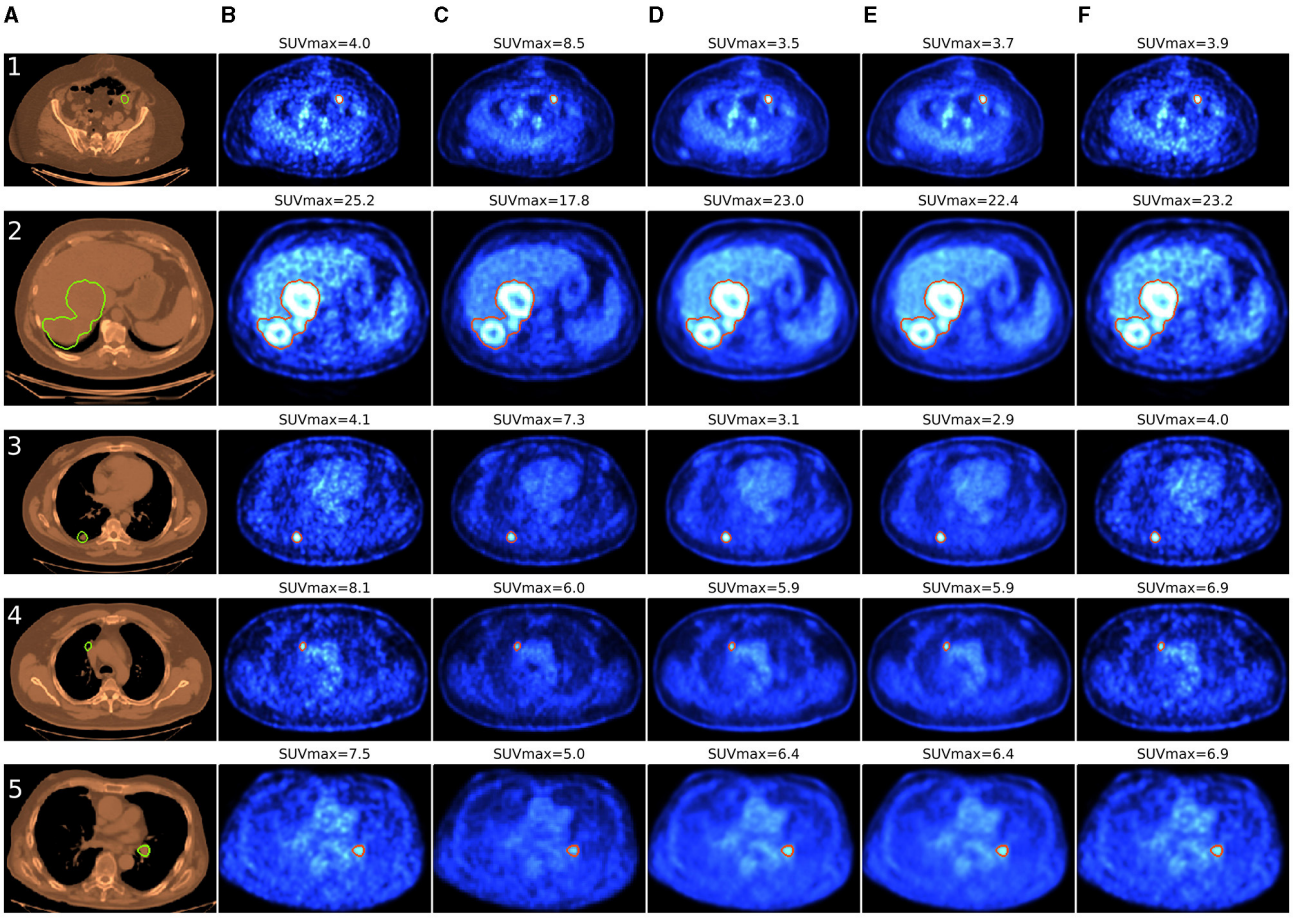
Comparison of denoising methods applied on low-time (30 s) PET reconstruction and SUVmax estimation within the region of interest. Tumor segmentation is done automatically by nnUnet using CT and PET. (A) CT (B) Low-time PET (30 s). (C) Full-time PET (90 s). (D) Reconstructed PET (by SwinIR 5 layers). (E) Reconstructed PET (by MedNeXt small). (F) Reconstructed PET (by Gaussian filter).
